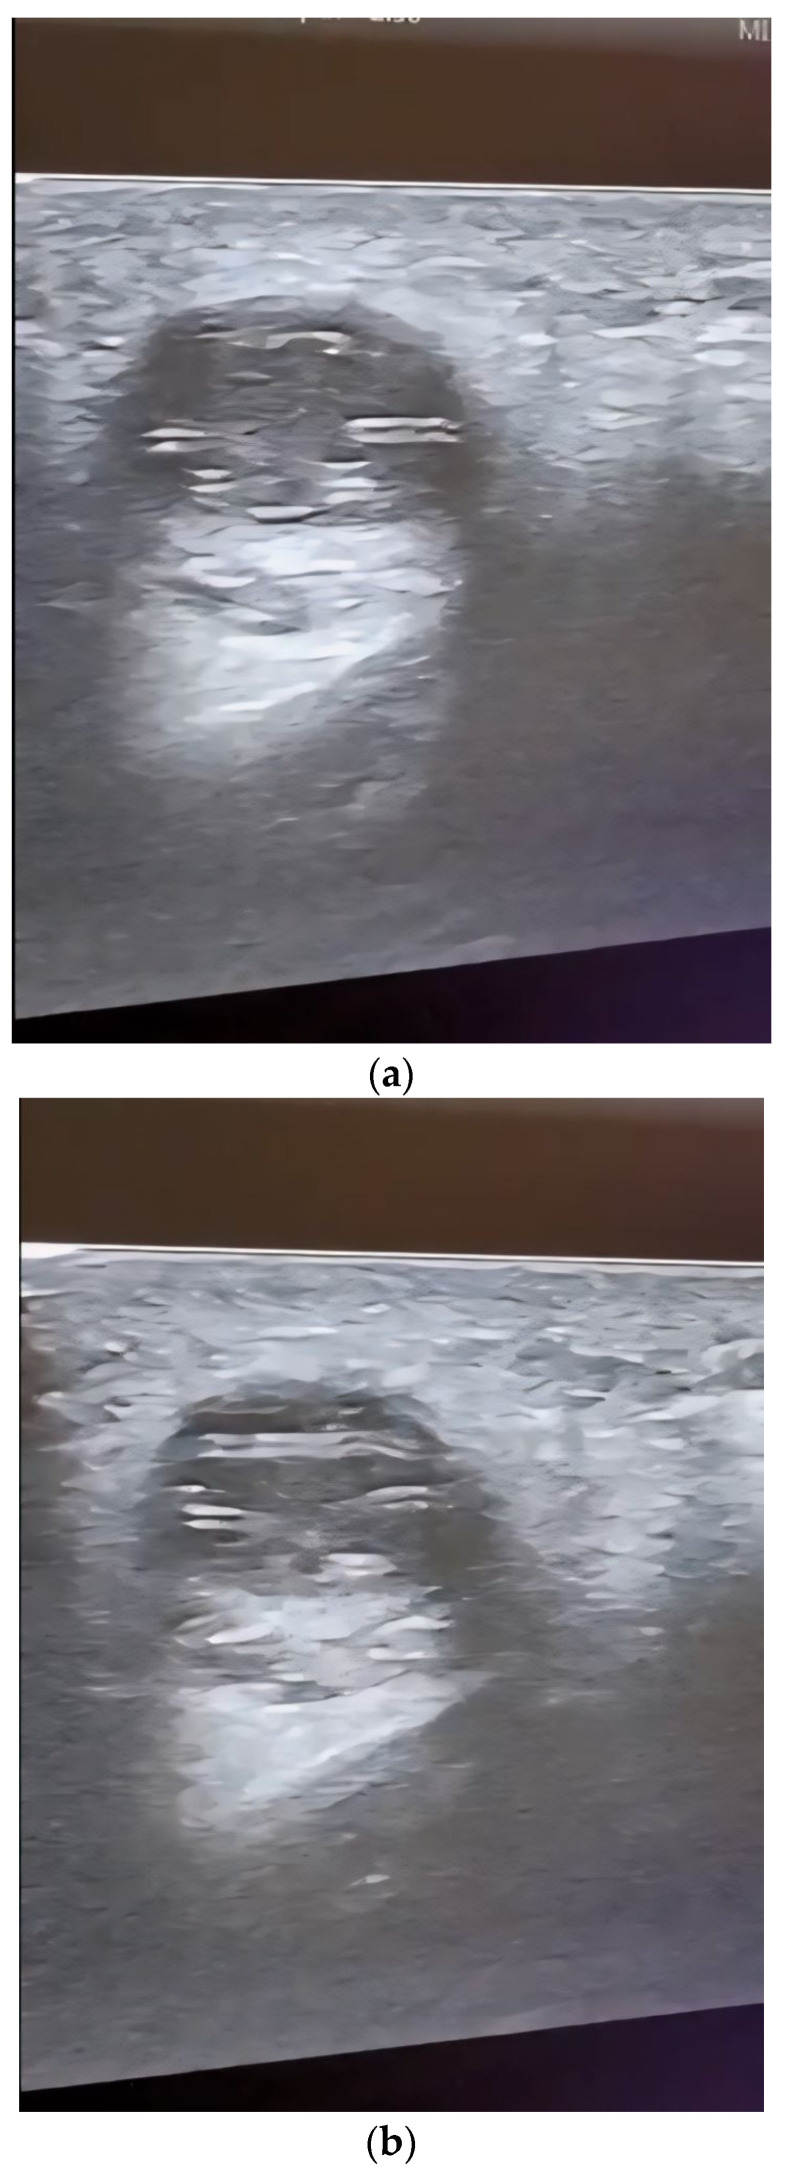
(a,b) Ultrasound image of the genian region showing a cystic mass including a motile tubular structure in different positions: (a) position 1; (b) position 2.
